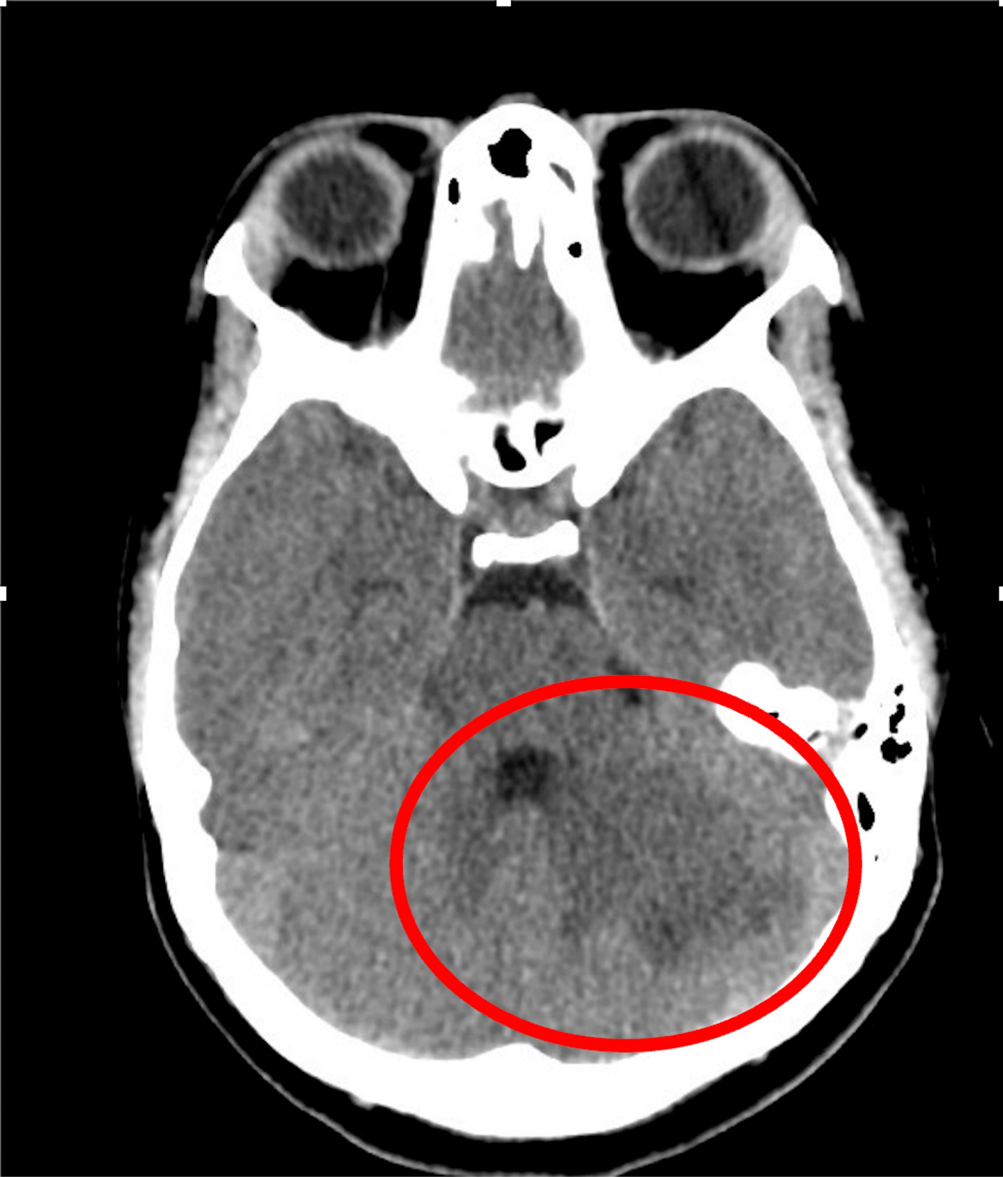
CT of the brain, axial view, showed an area of hypo-density in the left cerebellar hemisphere in the supero-lateral aspect, representing an infarct.
